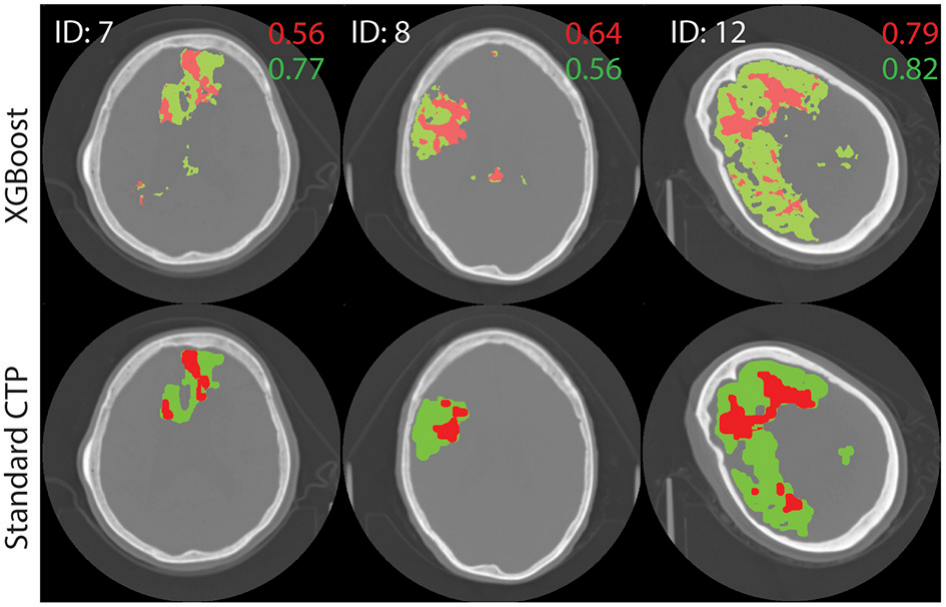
Test image results. A single axial slice, selected to clearly display the lesion, is shown from each image. The results of processing test images through the XGBoost model to make a prediction on the class label is shown at top. Standard lesion maps are shown at bottom. The predictions for core (red) and penumbra (green) are shown on top of a single axial slice of the brain, obtain with non-contrast CT. Dice similarity score are shown on the image, in corresponding colors.
